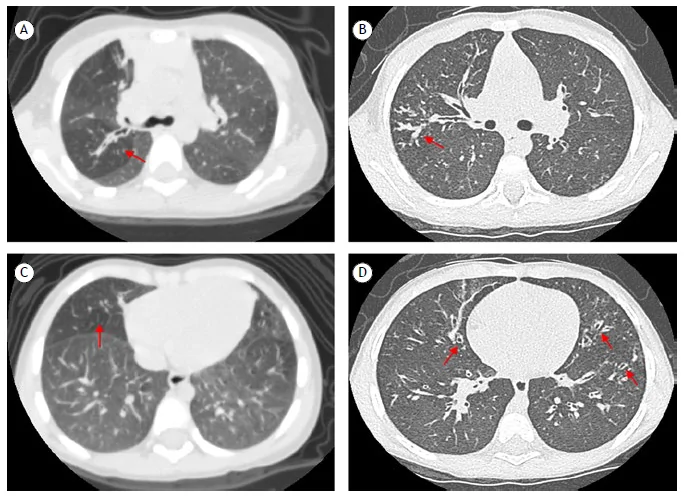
Chest CT findings in two pediatric cystic fibrosis patients at 3 years of age. In A, regional air trapping and bronchiectasis. In B, middle lobe air trapping. In C, mucoid impaction. In D, bronchiectasis. All findings are indicated by red arrows.
